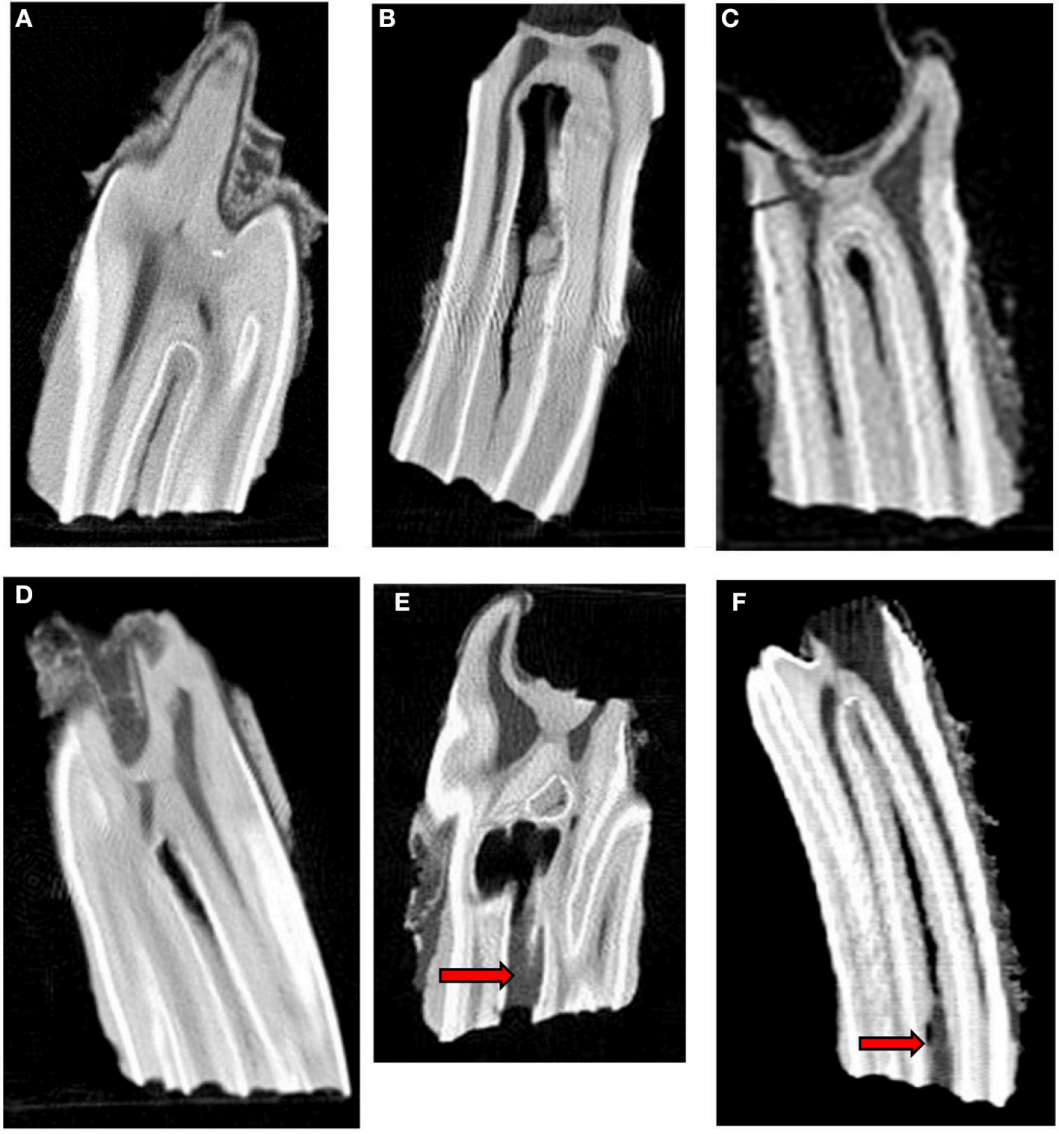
Longitudinal standard CT images of 6 infundibular lesions. (A) Longitudinal CT image of an older tooth showing a fine central linear cemental defect. This defect appears slightly wider than the “normal” central vascular channel. (B) Longitudinal CT image of a young tooth shows a central linear cemental defect (2–5 mm wide) continuous with marked apical cemental hypoplasia. There is no occlusal cemental defect and consequently no subocclusal caries at this stage. (C) Longitudinal CT image of an older tooth showing localized apical cemental hypoplasia extending occlusally as a linear type central defect to about 50% of infundibular depth (D) Longitudinal CT image of an older tooth shows localized apical cemental hypoplasia/aplasia, with a thin oblique layer of hypoplastic cement between it and the normal appearing cementum. (E) Longitudinal CT image of an older tooth. Occlusal caries is continuous with extensive subocclusal infundibular caries (arrow) and to an apical diverticulum with marked/total cemental hypoplasia that would later become filled with food. (F) Longitudinal CT image of a young tooth showing a wide hypointense, central linear cemental defect connected to an occlusal caries lesion (arrows) of soft tissue density.
